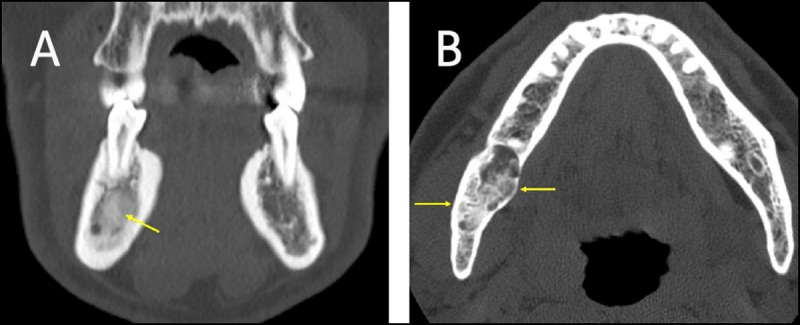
(A) Condensing osteitis in two patients. (a) Coronal reformation CT in a patient with history of right-sided periapical inflammation and abscess formation. A poorly marginated nonexpansile sclerotic lesion is seen (arrows). (B) Axial CT image obtained in a patient with longstanding caries who underwent tooth extraction. A poorly defined sclerotic lesion is seen adjacent to the extraction site (arrows).
